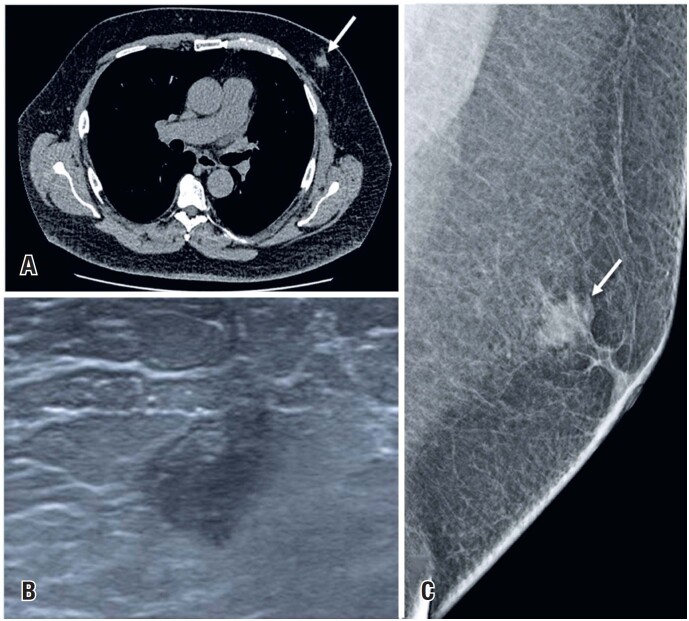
A 63-year-old man underwent a chest CT for a chronic cough. (A) The CT scan incidentally identified an irregular mass in the left breast (arrow). (B) On ultrasound showed the hypoechoic irregular mass. (C) Mammography showed an irregular indistinct mass—*BI-RADS 4*. The biopsy confirmed *invasive ductal carcinoma*
